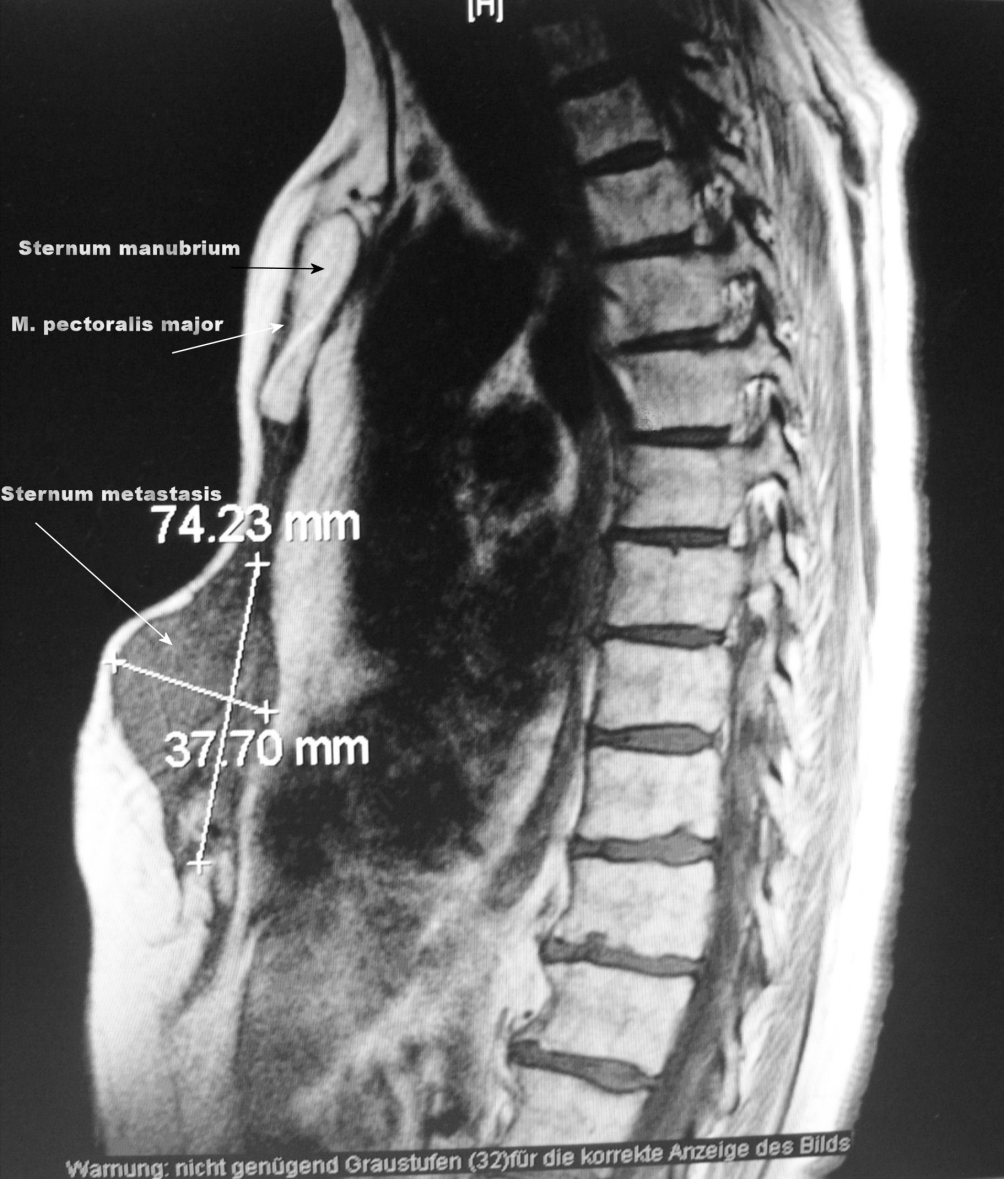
Magnetic resonance imaging of the sagittal plan of the large adenocarcinoma. Whole thickness invasion of the sternum, surrounding fat tissues, and the anterior mediastinum is shown.
